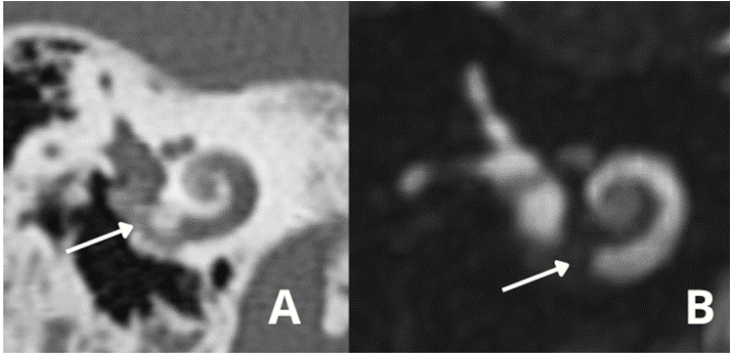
(A) Obliteration of the round window and part of basal turn.A ‒ Temporal bone high-resolution computed tomography; (B) MRI ‒ T2-weighted sequence.
